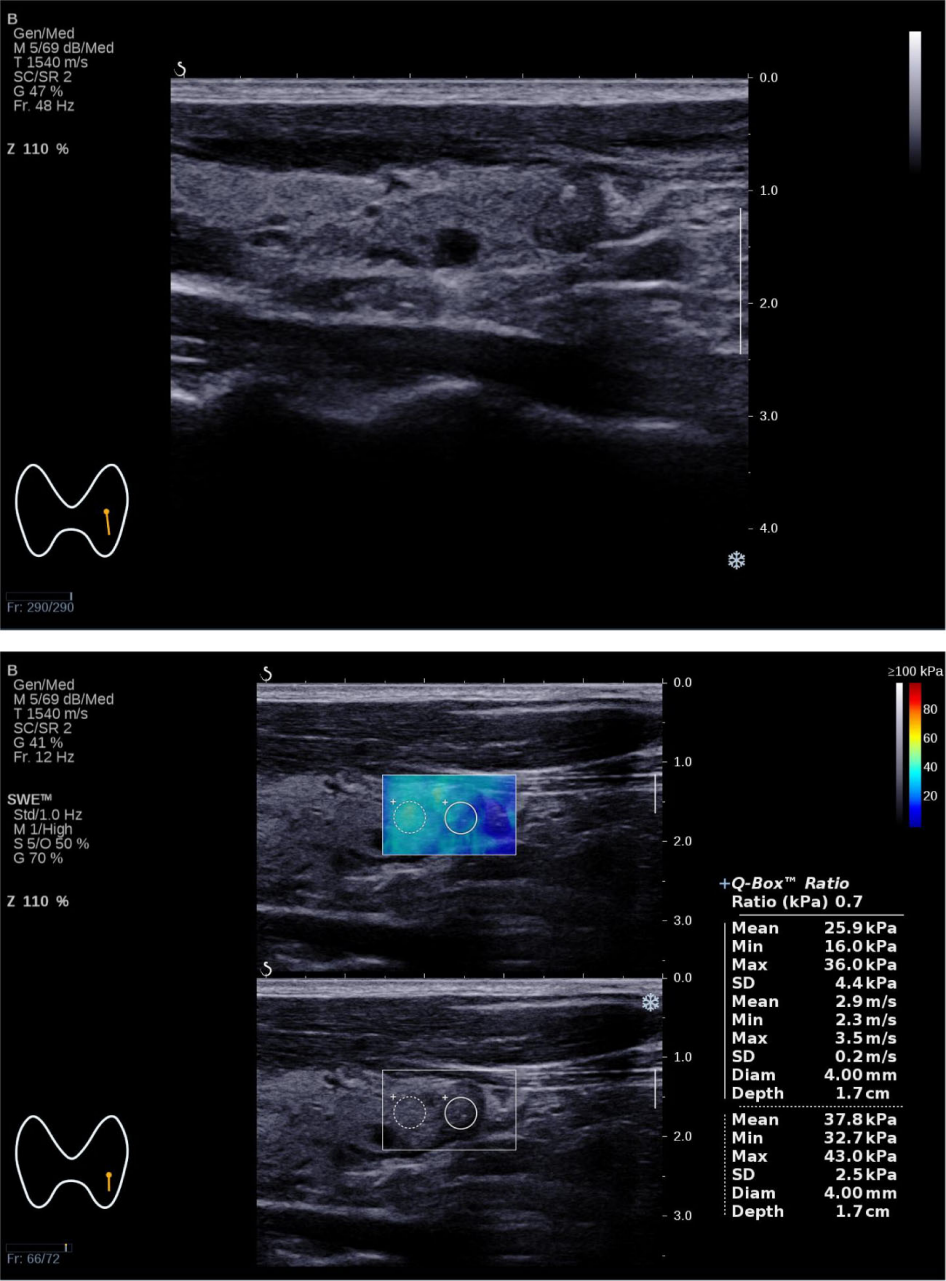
The Bethesda I thyroid nodules of a 31-year-old man was predicted with the combined model. A 31-year-old man with a 7×6×6 mm thyroid nodule in the left lobe. Conventional ultrasound showed a solid hypo-echoic, regular nodule taller than wider with coarse calcifications, which was categorized as C-TIRADS 4B. SWE measurement showed that *E*
